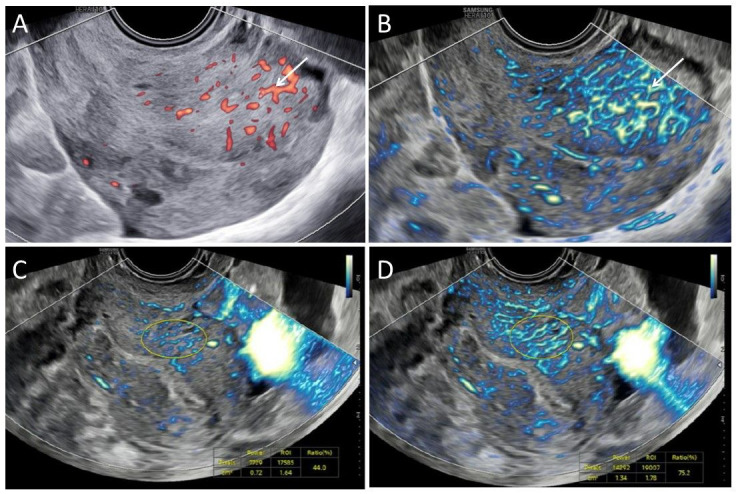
Representative images of a uterus with adenomyosis and influence of cardiac cycle. A. Adenomyosis in power Doppler mode; B. Adenomyosis in MV-flow^TM^ mode. Arrow indicates translesional flow in adenomyotic lesion; C. Adenomyosis visible in diastole, two dimensional (2D) microvascular flow vascular index 44,0; D. Adenomyosis visible in systole, 2D microvascular flow vascular index 75,2.
